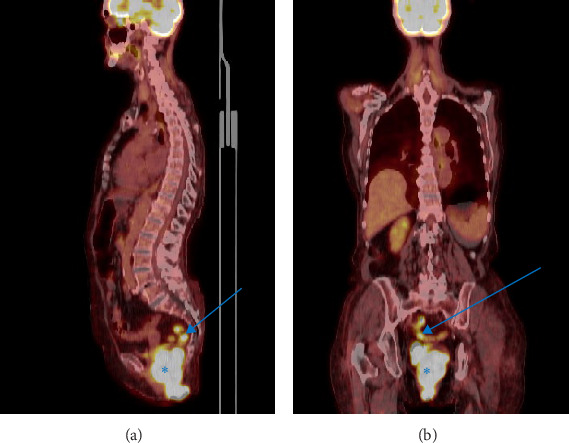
A PET scan superimposed on a CT scan. Image A –a sagittal view. Image B– a coronal view. Blue arrow points to the sigmoid colon with a mildly elevated glucose metabolism −11.56 g/mL standardized uptake value (SUV). Blue “*⁣*^*∗*^” symbol marks the rectal tumor with an elevated glucose metabolism −23.58 g/mL SUV. The elevated glucose metabolism was attributed to the inflammatory process, with no suspicion of malignancy raised. No lymph nodes with increased glucose metabolism were found.
